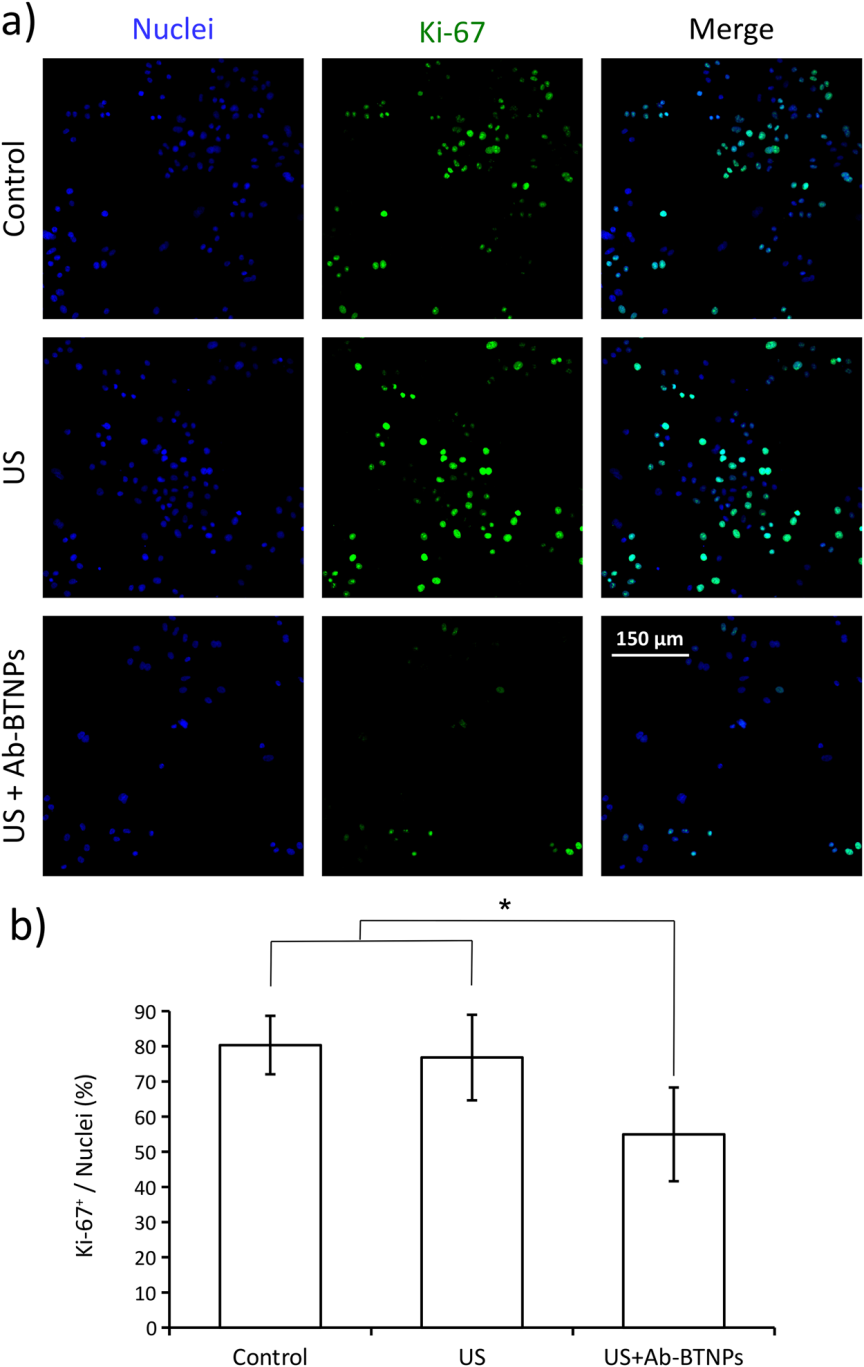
Effects of the chronic piezoelectric stimulation (US + Ab-BTNPs) on Ki-67 expression. (a) Confocal fluorescence images showing Ki-67 expression on control cultures, on ultrasound-stimulated cells without Ab-BTNPs (US), and on ultrasound-treated cultures after incubation with Ab-BTNPs (US + Ab-BTNPs); Ki-67 is shown in green, nuclei in blue. The percentages of Ki-67^+^ nuclei for each condition are shown in (b). p* < 0.05.
